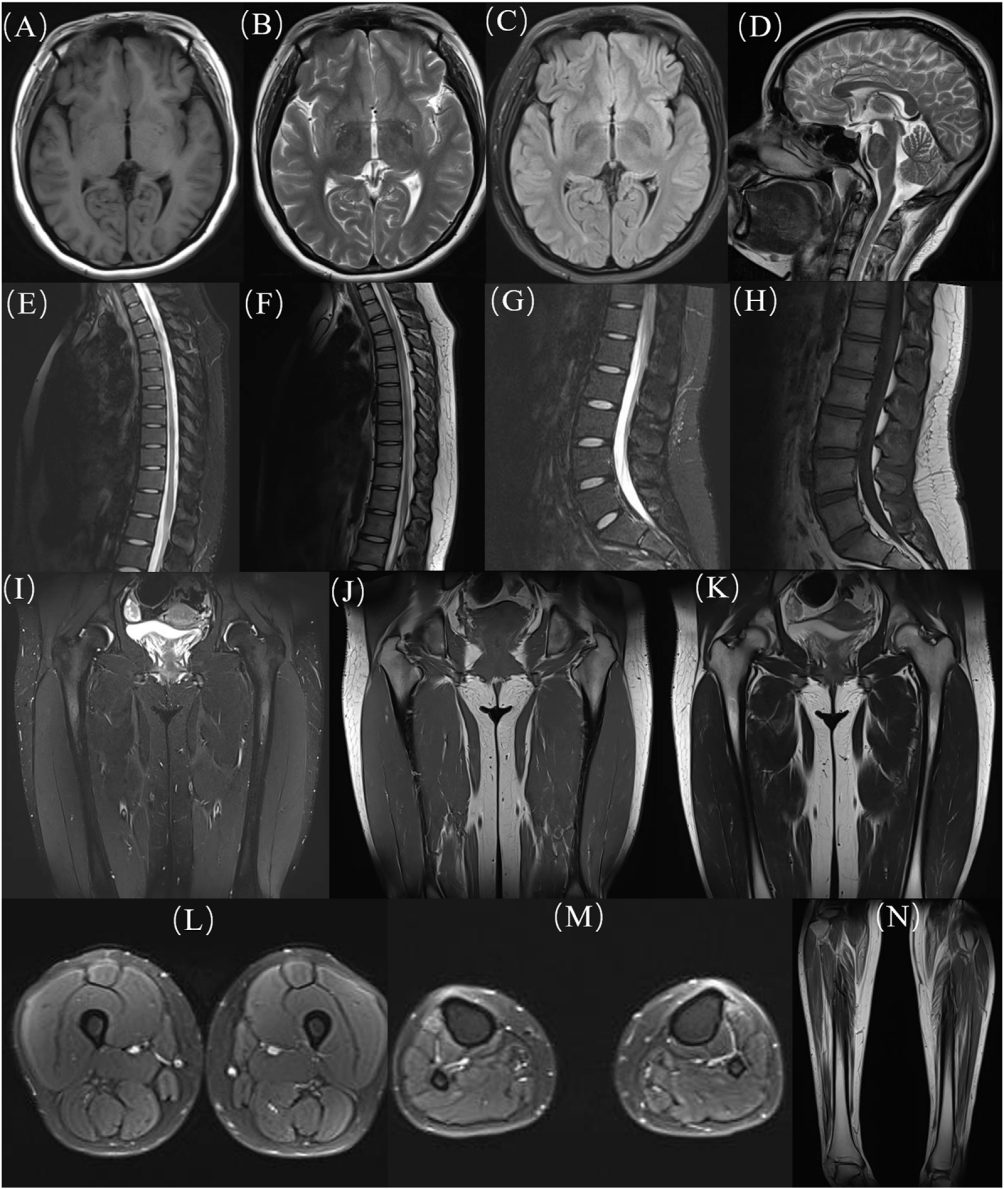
MRI images of the patient. (A-D) head, (E, F) thoracic segments; (G, H) lumbar segments; (H-K) hips; (L) thighs; (M, N) calves. MRI: Magnetic resonance imaging.
